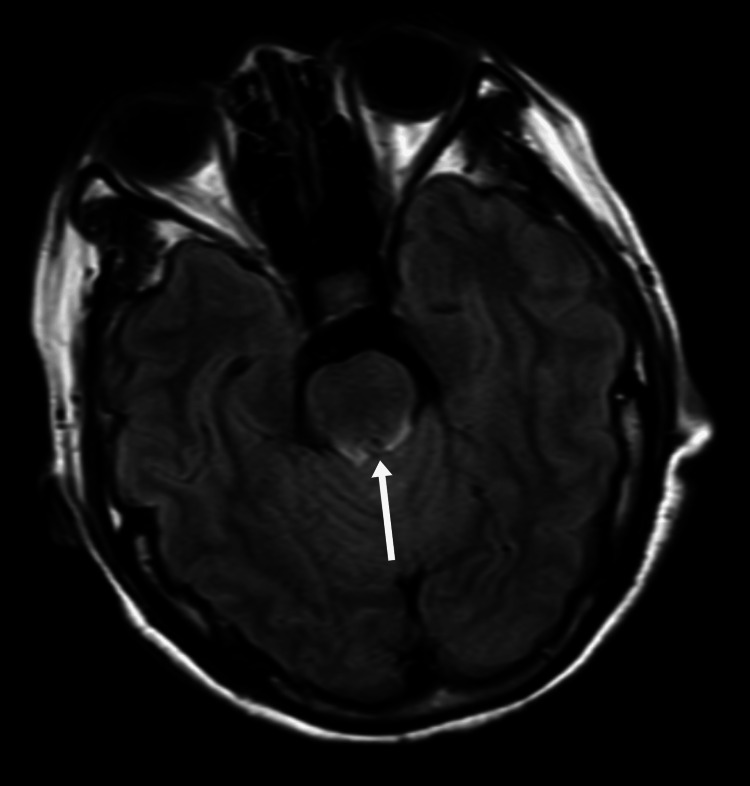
Axial FLAIR MRI of the cerebral peduncle before treatment As indicated by the white arrow, there is an abnormally high signal in the cerebral peduncle FLAIR: fluid-attenuated inversion recovery; MRI: magnetic resonance imaging
